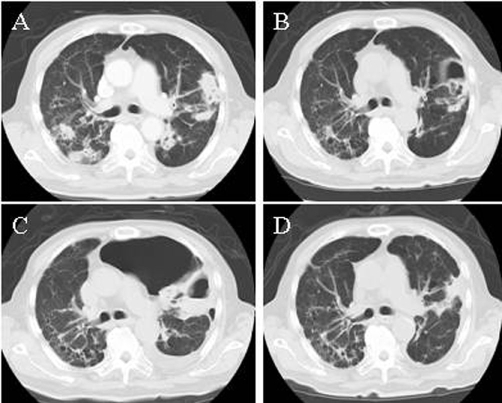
Chest computed tomography scans. Chest computed tomography scans on admission (A), 3 (B), 6 (C), and 12 weeks (D) after the onset of the induction therapy.
